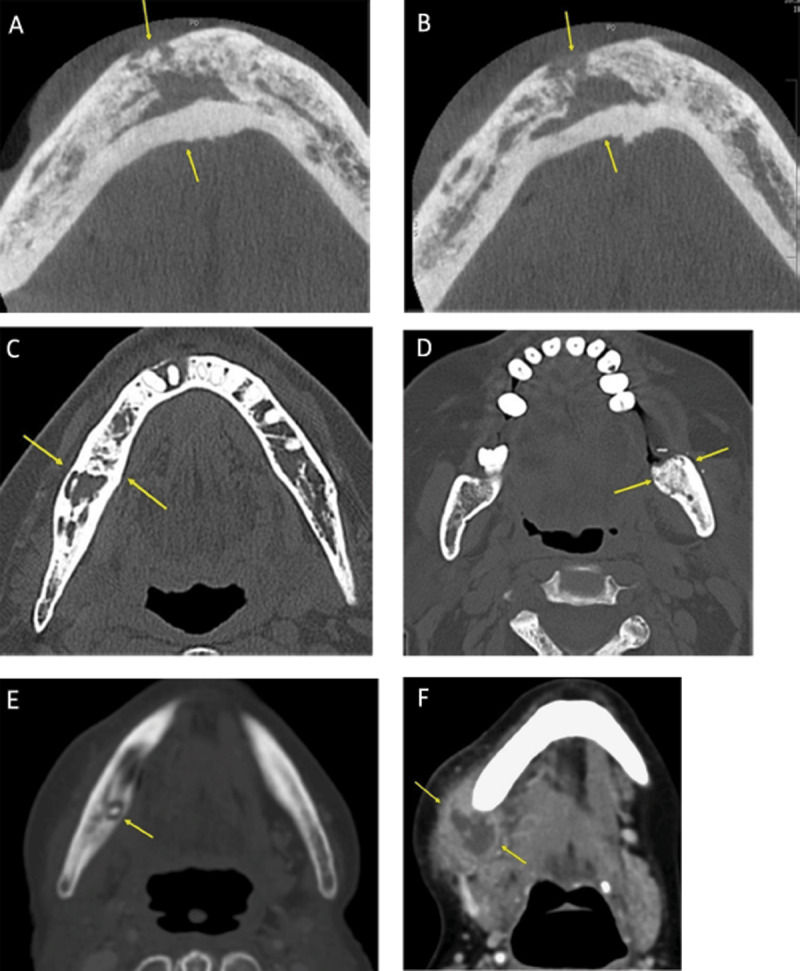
(A, B) Mandibular osteoradionecrosis. Axial CT images showing extensive areas of mandibular sclerosis with a loss of trabeculation in spongiosa, and cortical interruptions (arrows). (C, D) Bisphosphonate-related osteonecrosis of the jaw (BRONJ) in 2 different patients. Axial CT images shows predominant right-sided sclerosis with interspersed osteolytic areas (c), and predominant left-sided sclerosis with pathologic fracture (d) (arrows). (E, F) Mandibular osteomyelitis in an elderly woman who underwent dental extraction. (e) Axial CT in bone window shows heterogeneous bone density of the right mandible with focal osteolysis and a small sclerotic sequestrum. (f) Contrast-enhanced axial CT image in soft tissue window shows an associated submandibular abscess (arrows).
